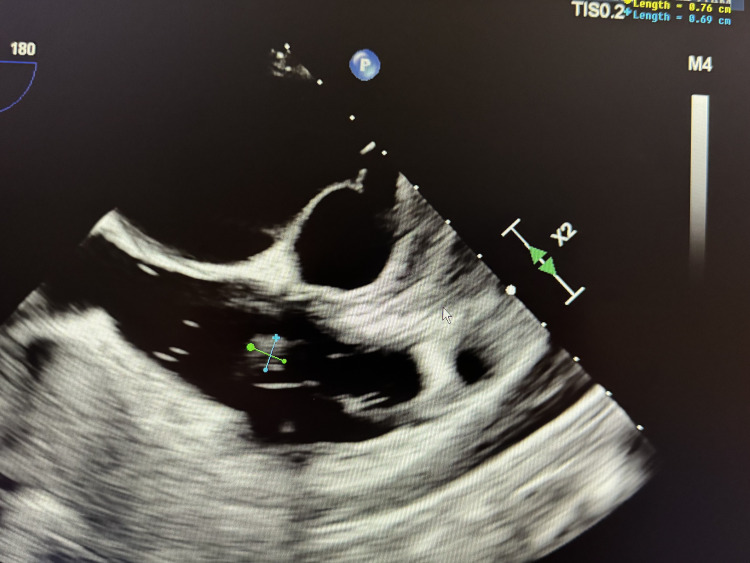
Transesophageal echocardiogram; the marked area shows vegetation in the tricuspid area that is pathognomonic for infective endocarditis.
